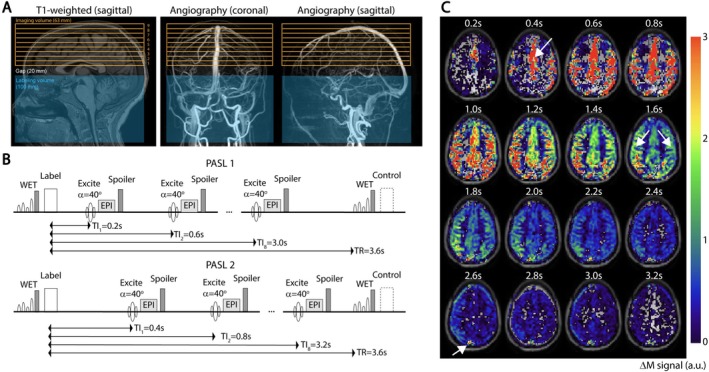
MRI acquisition and example single participant data. (A) The imaging volume is planned with the most distal slice above the most distal aspect of the superior sagittal sinus, with coverage extending proximally over the majority of the bi‐frontal and bi‐parietal lobes, where infarcts are most common in patients with sickle cell anemia. Imaging and labeling volumes are overlaid on *T*
